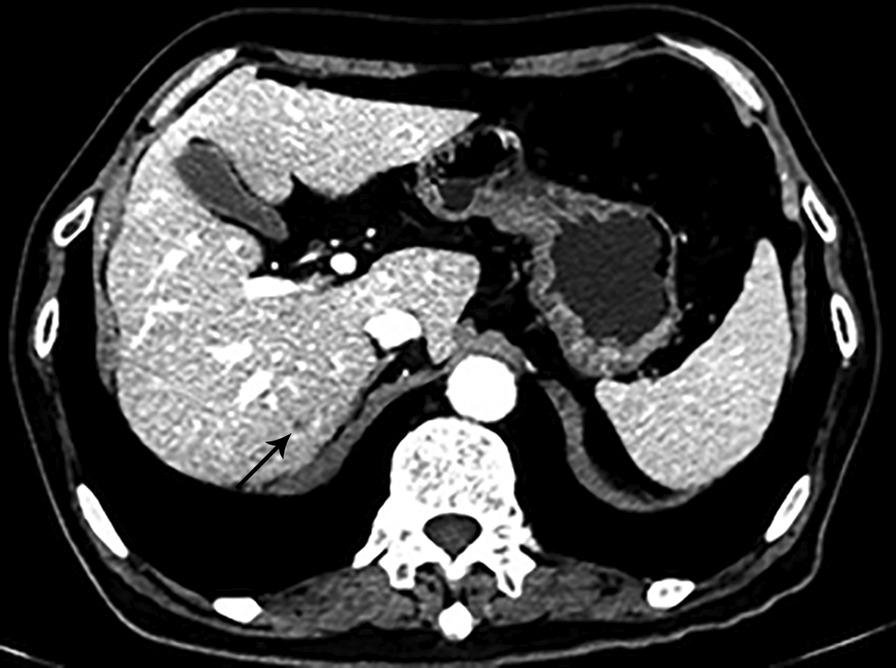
Abdominal CT. After enhancement, abdominal CT shows a small low-density lesion in the seventh segment of the liver (black arrow)
